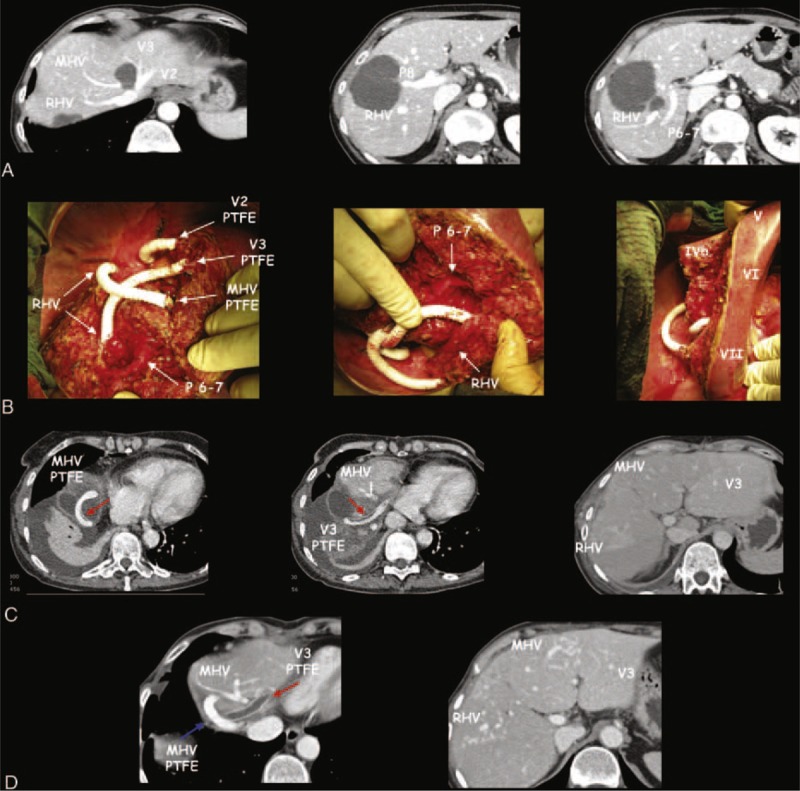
A case of minor-*but*-complex hepatic resection with hepatic vein reconstruction with ringed PTFE graft used as “a bridge” to intrahepatic shunt maturation. This patient had 6 synchronous colorectal metastasis (CRLM) and after the resection of primary tumor had good response to 8 cycles of FOLFOXIRI plus Bevacizumab. (A) This case was judged potentially inoperable on the basis of preoperative CT scan. Liver outflow was infiltrated by different CRLM at the hepato-caval confluence (CC) involving the left hepatic vein (HV) for segments 2 and 3 (V2, V3) and middle HV (MHV), and far from the CC involving the right HV (RHV). Liver inflow was also involved by CRLM interesting right portal branch for segment VIII (P8) and the CRLM infiltrating RHV close to the posterior right portal branch (P6–7). (B) The intraoperative field of the resection of segment VIII–IVa partially extended to segment VII and II and to the paracaval portion of segment I with the exposure of all the main intrahepatic vascular structures at the CC. Left hepatic outflow was reconstructed with 3 ringed PTFE grafts: V2 was reconstructed directly on the cava vein, V3 was reconstructed on the RHV where it was tangentially resected for the adjacent CRLM, the MHV was reconstructed at the level of the confluence of the hepatic vein of segment VII with the RHV. Right inflow was preserved with the complete exposure of P6–7 and with the section of P8 at his origin. The resected liver presented no sign of congestion with all the residual segments well perfused. The liver cut surface was 241 cm^2^, the cumulative clamping time was 292 minutes, 8 units of red packed cells were requested (preoperative anemia 8,6 Hb and metabolic acidosis due to chemotherapy), the postoperative course was characterized by transient ascites, and the patient was discharged on the 14th postoperative day; successively, a delayed biloma was treated with a percutaneous drainage. Eight months after liver surgery the patient is alive and well without any sign of disease recurrences. (C) The CT scan on the 11th postoperative day shows a well perfused left liver in spite of the thrombosis of the V2 PTFE graft (due to presence of intrahepatic shunts between V2 and V3). There were no-evident shunts between V3 to MHV and MHV to RHV. Red arrows indicate a laminar thrombus in both MHV and V3 PTFE. (D) The CT scan 5 months after shows the resolution of the thrombus in the MHV PTFE graft (blue arrow) and the complete thrombosis of the V3 PTFE graft (red arrow) with a well perfused left liver because of the spontaneous maturation of peripheral shunts between V3 and MHV; peripheral shunts are also maturating around RHV.
